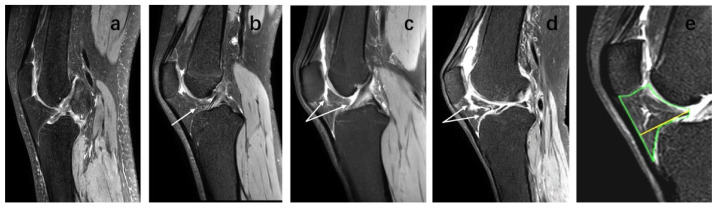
IPFP signal intensity alteration, IPFP maximum cross-sectional area (CSA) and IPFP depth on sagittal planes of T2-weighted images. IPFP signal intensity alteration graded as follows: (a) Grade 0 = none; (b) Grade 1 ≤ 10% of the area; (c) Grade 2 = 10–20% of the area; (d) Grade 3 ≥ 20% of the area. The area indicated by the white arrow within the IPFP represent the high signal regions. (e) The green area indicates the IPFP maximum CSA. The yellow line which perpendicular to the patellar tendon was drawn from the anterior to posterior surface indicates IPFP depth.
